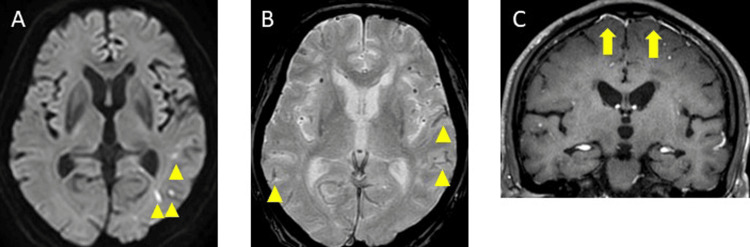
Brain contrast magnetic resonance imaging (MRI) and magnetic resonance angiography (MRA) on day 66 A: Diffusion-weighted imaging showed an increase in small high signals in the left parietal occipital lobe (yellow triangle). B: T2* imaging demonstrated an increase in cortical superficial siderosis in the right parietal, left frontal, and parietal sulcus (yellow triangle). C: A contrast-enhanced brain MRI on day 66 indicated contrast enhancement in the meninges (yellow arrow).
